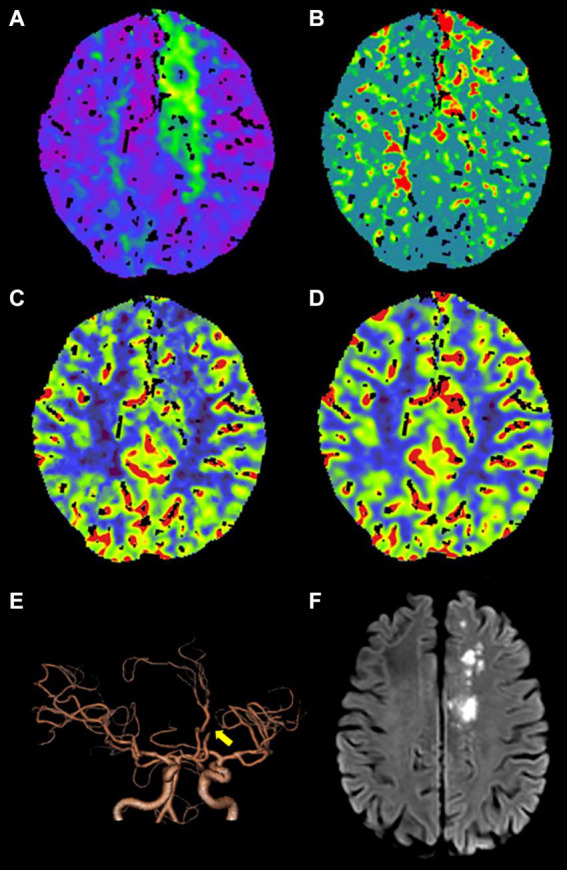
An adult patient with TIA presented with secondary cerebral infarction within 90 days of follow-up. (A–D) CTP maps, including time-to-maximum (Tmax), mean transit time (MTT), cerebral blood flow (CBF), and cerebral blood volume (CBV), reveal that Tmax is prolonged (A), MTT is prolonged (B), CBF is slightly decreased (C), and CBV exhibits no obvious change (D) in the left frontal lobe and centrum semiovale. (E) Computed tomography angiography (CTA) indicates occlusion of A2 segment of the left anterior cerebral artery (yellow arrow). (F) DWI shows the secondary cerebral infarction in the left frontal lobe and centrum semiovale within 90 days of follow-up.
